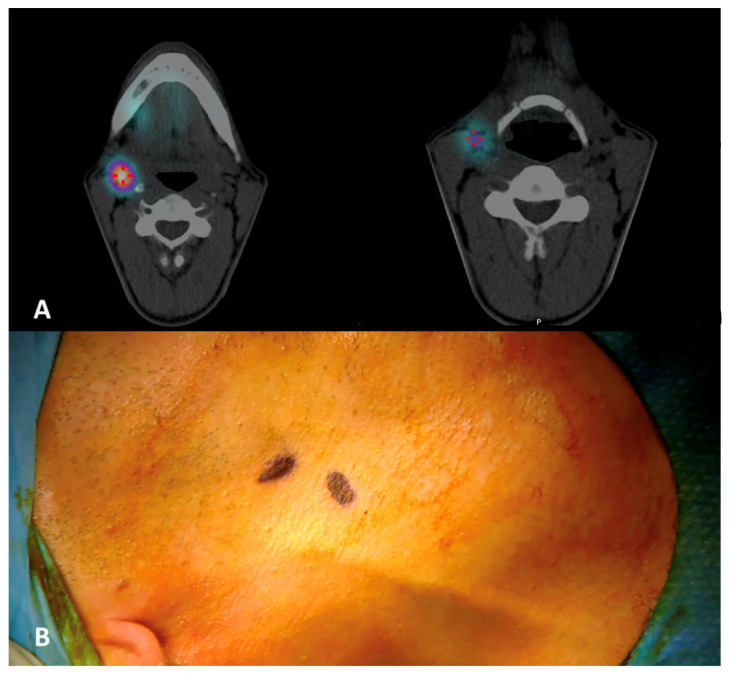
(A) Preoperative SPECT/CT with identification of two nodal hotspots (lighter circular areas encircled by a purplish ring) at levels IIA on the right side, one more cranially (left) and one more caudally (right). (B) Skin landmarking.
